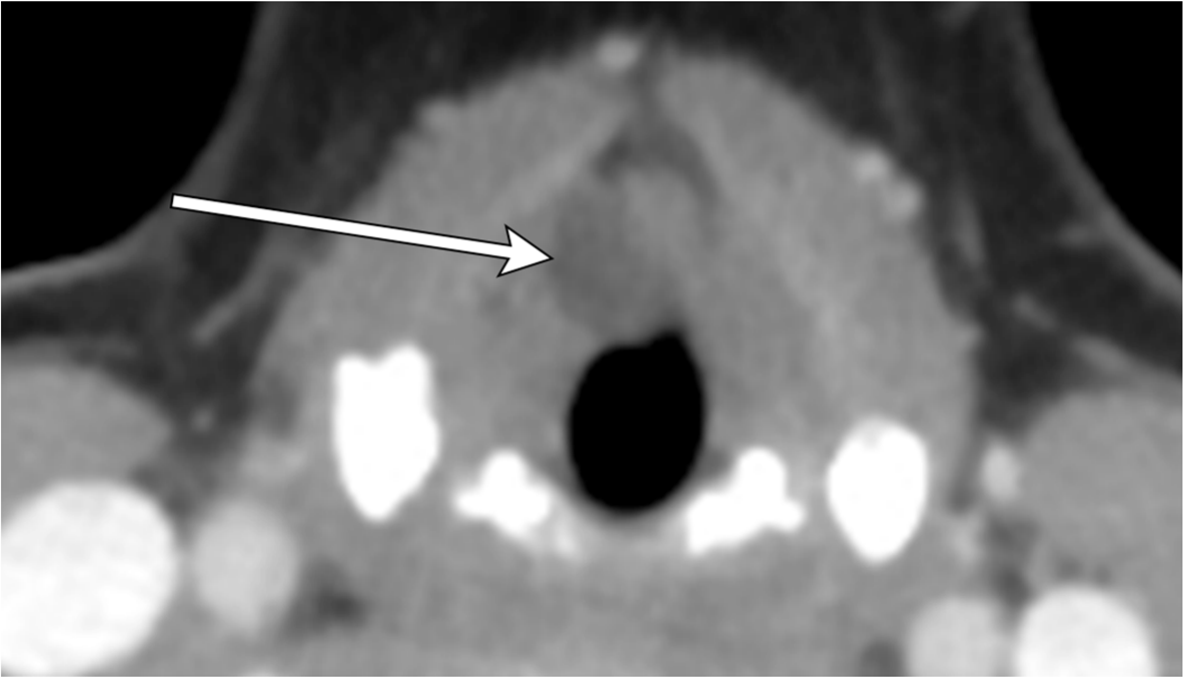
Internal laryngocele. Axial contrast-enhanced CT image shows a non-enhancing fluid attenuating structure in right paraglottic region with mass effect on false vocal cord (arrow)
